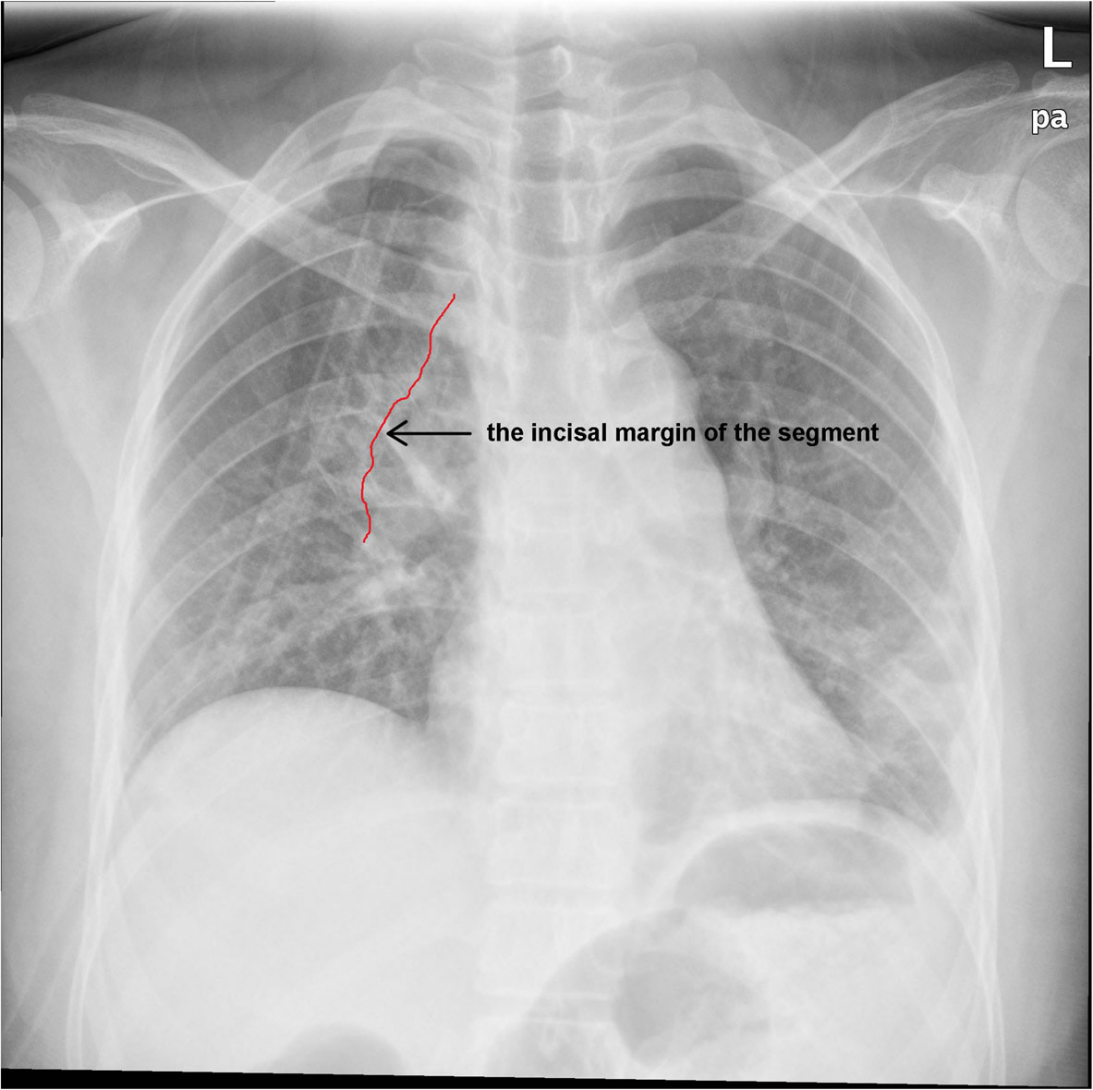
The chest radiograph on the 2nd postoperative day. The incisal margin of the segment displayed no obvious exudation (arrow)
